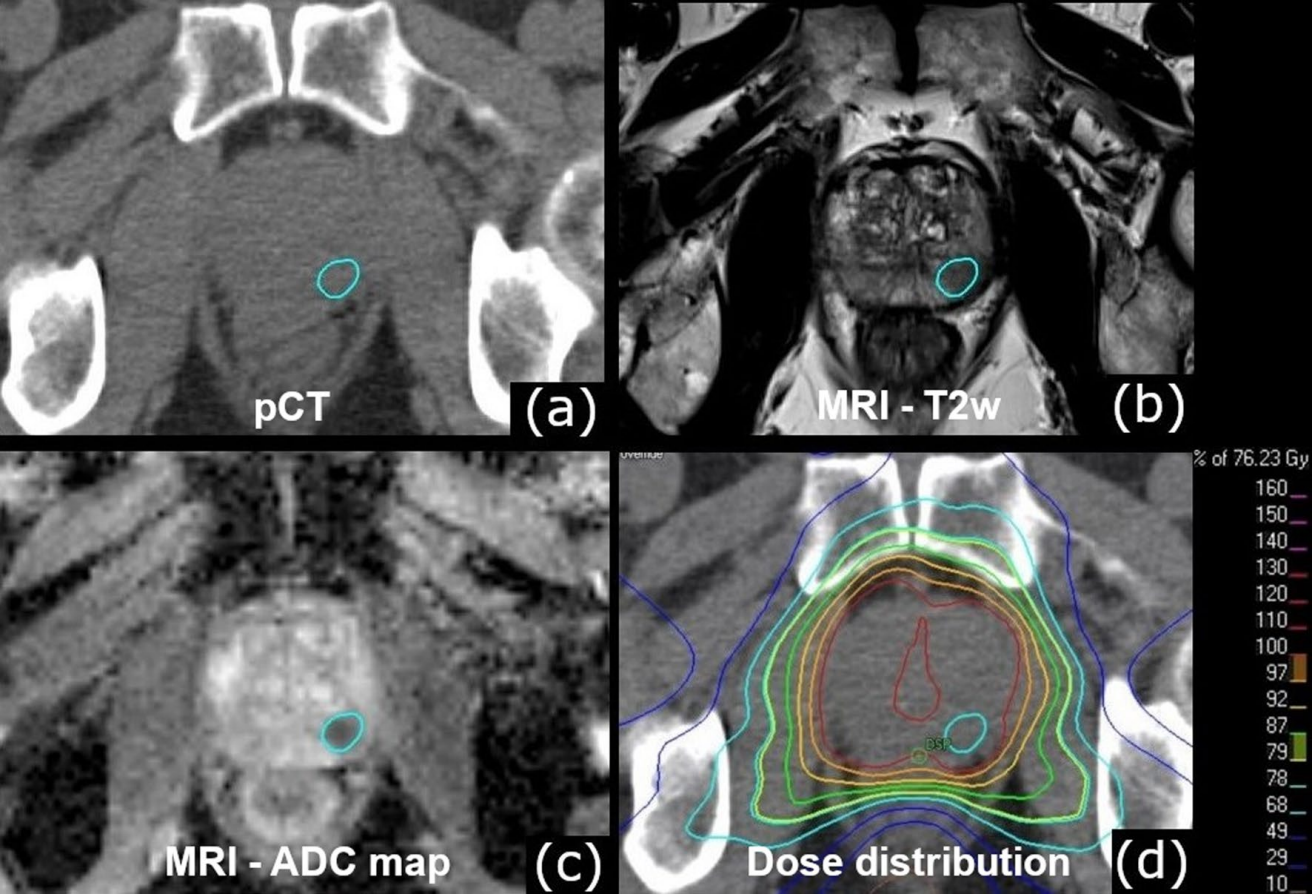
Dominant intraprostatic lesion contouring. Shown is the index lesion in the peripheral zone on the a planning CT, b native T2-weighted image, c apparent diffusion coefficient map, d planned dose distribution. Abbreviations: *pCT* planning CT, *MRI* Magnetic resonance imaging, *T2w* T2-weighted, *ADC map* apparent diffusion coefficient map
